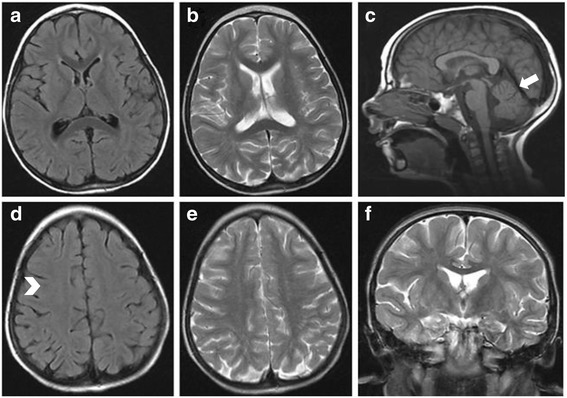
MRI of the patient at age 4 years. The images show very mild abnormalities of the white matter that are barely visible in FLAIR (a, d) (*arrow head*). Sagittal T1 weighted image shows a normal corpus callosum and a mild increased spacing of the cerebellar folia (*arrow*) (c). Coronal and axial T2 weighted images document peculiar fine stripes in T2 weighted image (b, e, f)
